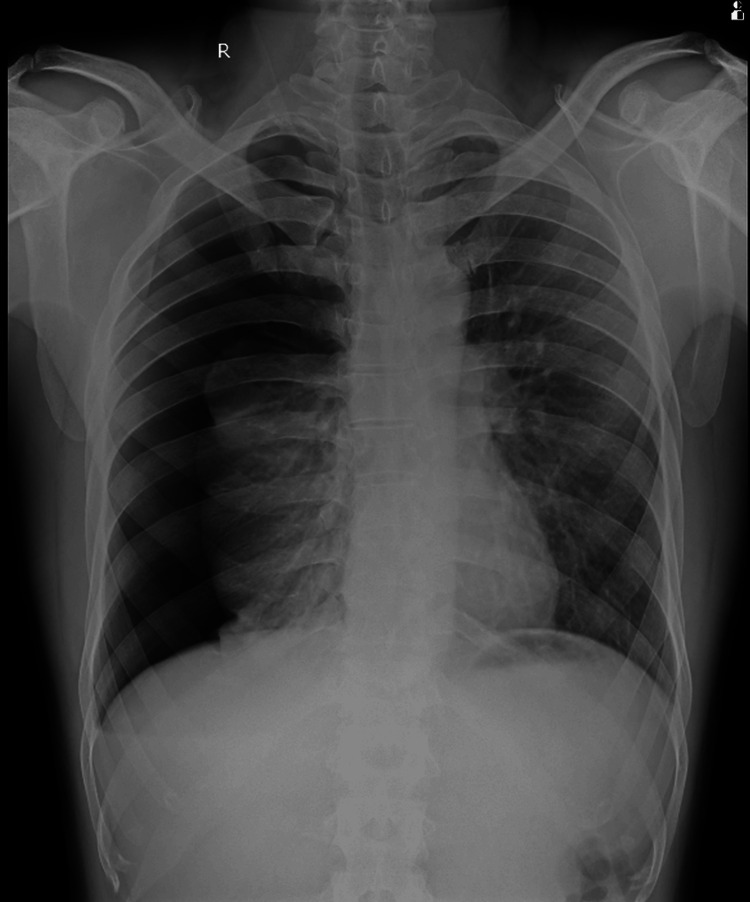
Chest X-ray (PA view) indicating a right-sided pneumothorax. PA, posteroanterior
